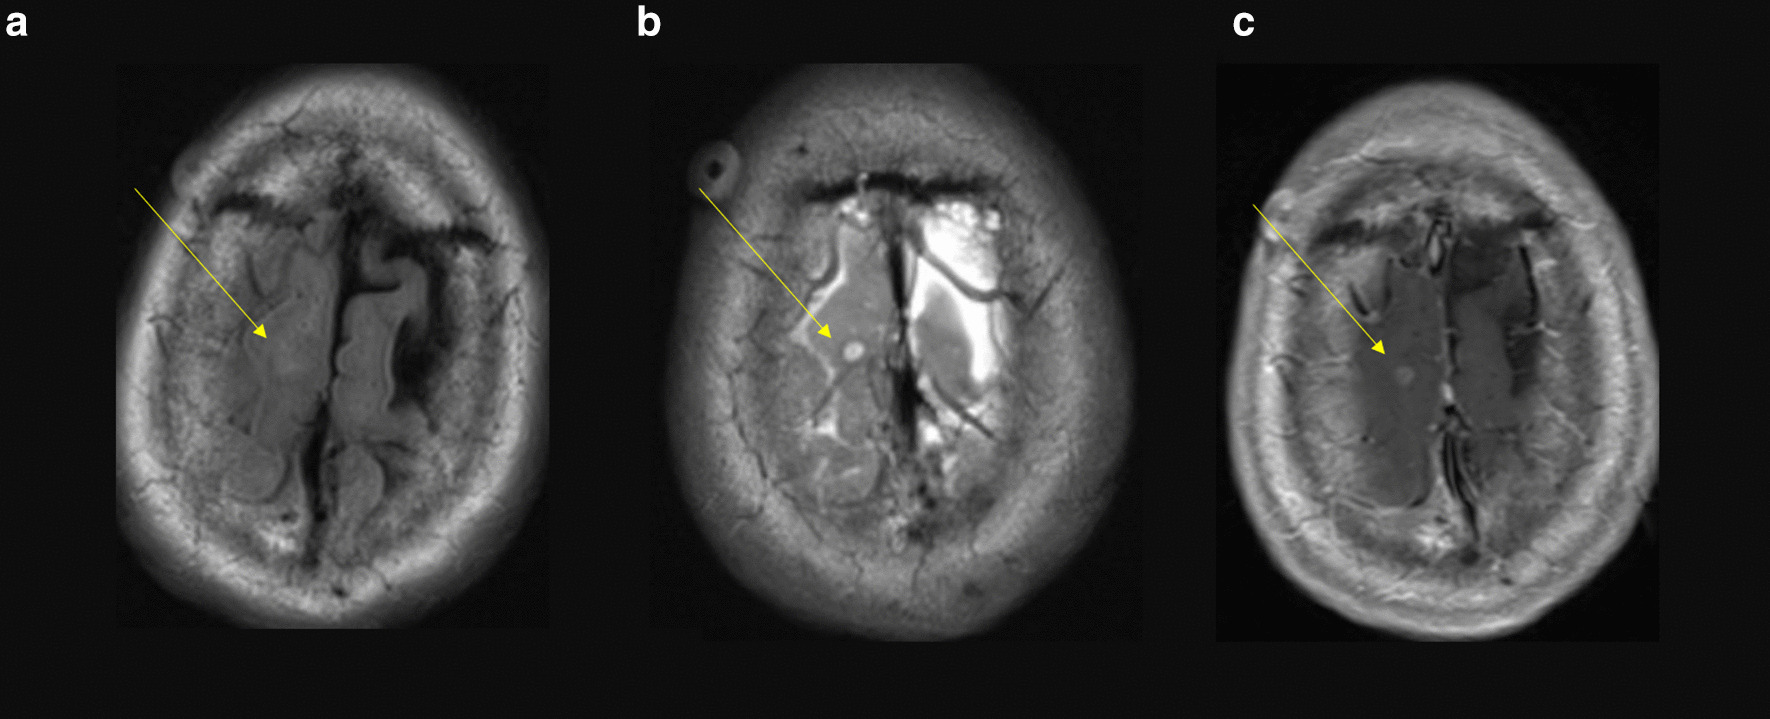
Fluid-attenuated inversion recovery (FLAIR) (a), T2 (b), and T1 (c) images demonstrating a 5 mm enhancing lesion (yellow arrow) in the right superior frontal gyrus
